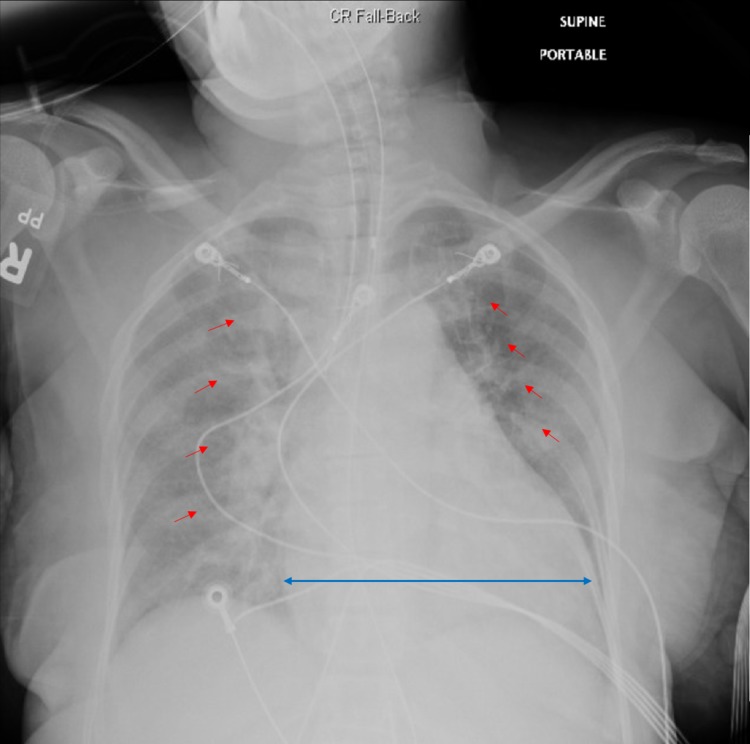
Post intubation chest X-ray showing increased bilateral airspace opacities which represent pulmonary edema (red arrows) and trace bilateral pleural effusions along with cardiomegaly (blue arrow)
